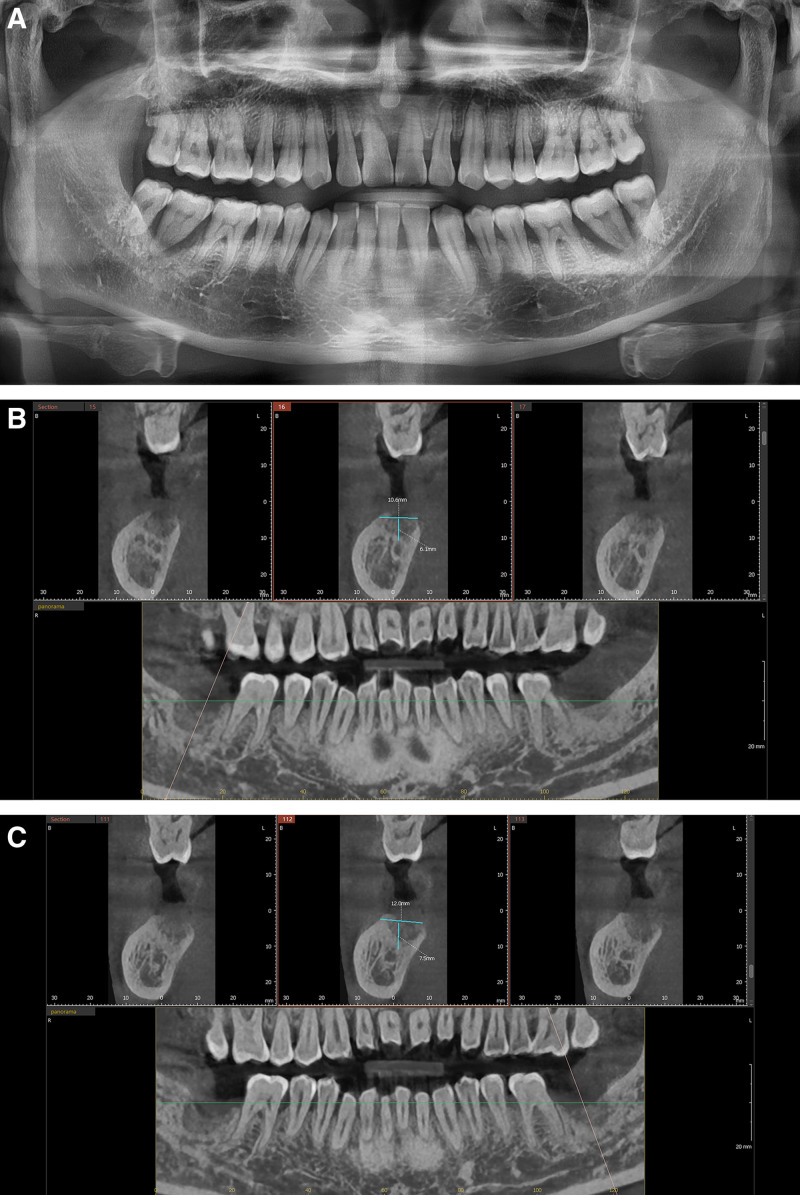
(A) Initial panoramic radiograph; (B) CBCT of site #47, 4 months post-extraction; (C) CBCT of site #37, 4 months post-extraction. CBCT = cone beam computed tomography.
